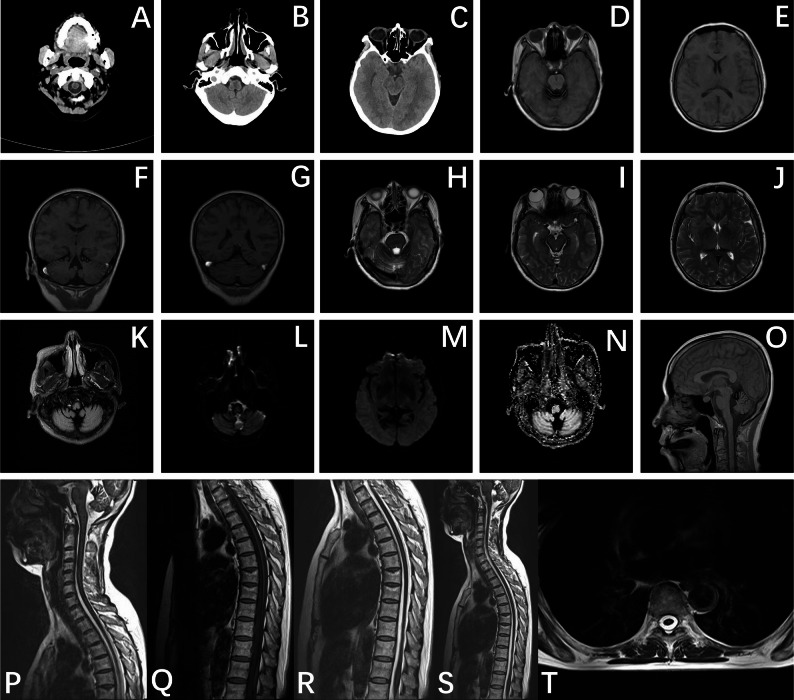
Case 5 A-C head CT: the surface of medulla oblongata, cerebellar hemispheres and around the fourth ventricle was covered with slightly higher linear signal; D, E, H-N cross-sectional head MRI, F-G coronal-section head MR: some sulcus gyrus widened and deepened, Cerebral stem and cerebellar atrophy, At the surface of the bilateral cerebral and cerebellar hemispheres sulci, cerebral split, cisterna and brainstem in the T1WI sequence, The T2WI, T2 Flair, DWI and SWI sequences are uniform linear sample with low signal shadow coverage; O-S MRI of sagittal head and cervicothoracic spine: spinal cord thinning, atrophy, enlargement of subarachnoid space, Brain stem and spinal cord surface in T1WI sequence, The T2WI sequence is a uniform line sample with low signal shadow coverage; graph T cross section thoracic vertebrae MRI T2WI: annular low signal shadow on the spinal cord surface
